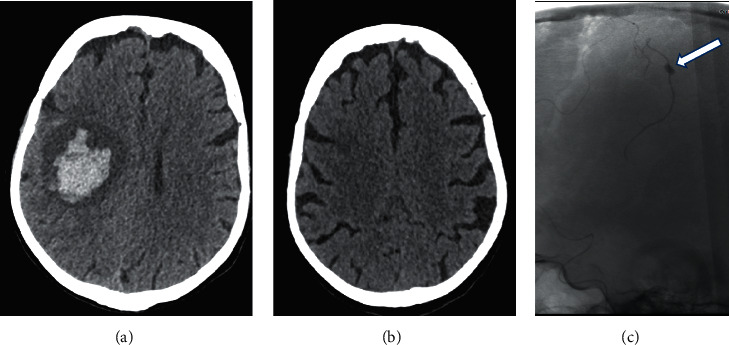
Hemorrhage from ruptured aneurysm right fronto-parietal hematoma (a); previously normal CT brain (b); angiography showing the aneurysm of a branch of the right middle cerebral artery (white arrow) (c).
